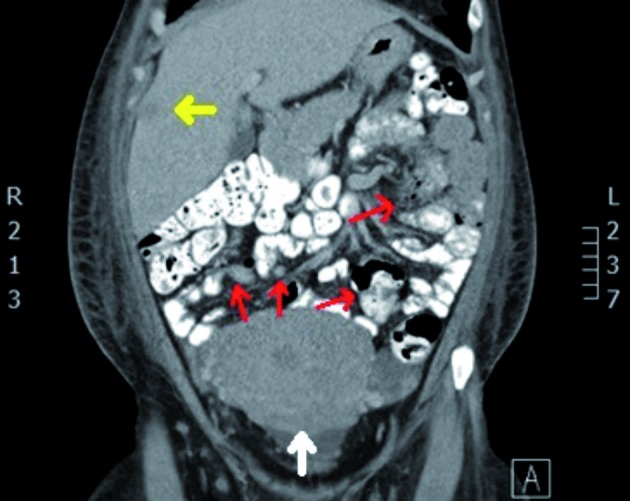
Abdominal CT scan: frontal section. Voluminous uterus (white arrow), liver metastasis (between lobe 5 and 8, yellow arrow) and mesenteric metastasis (red arrows).
